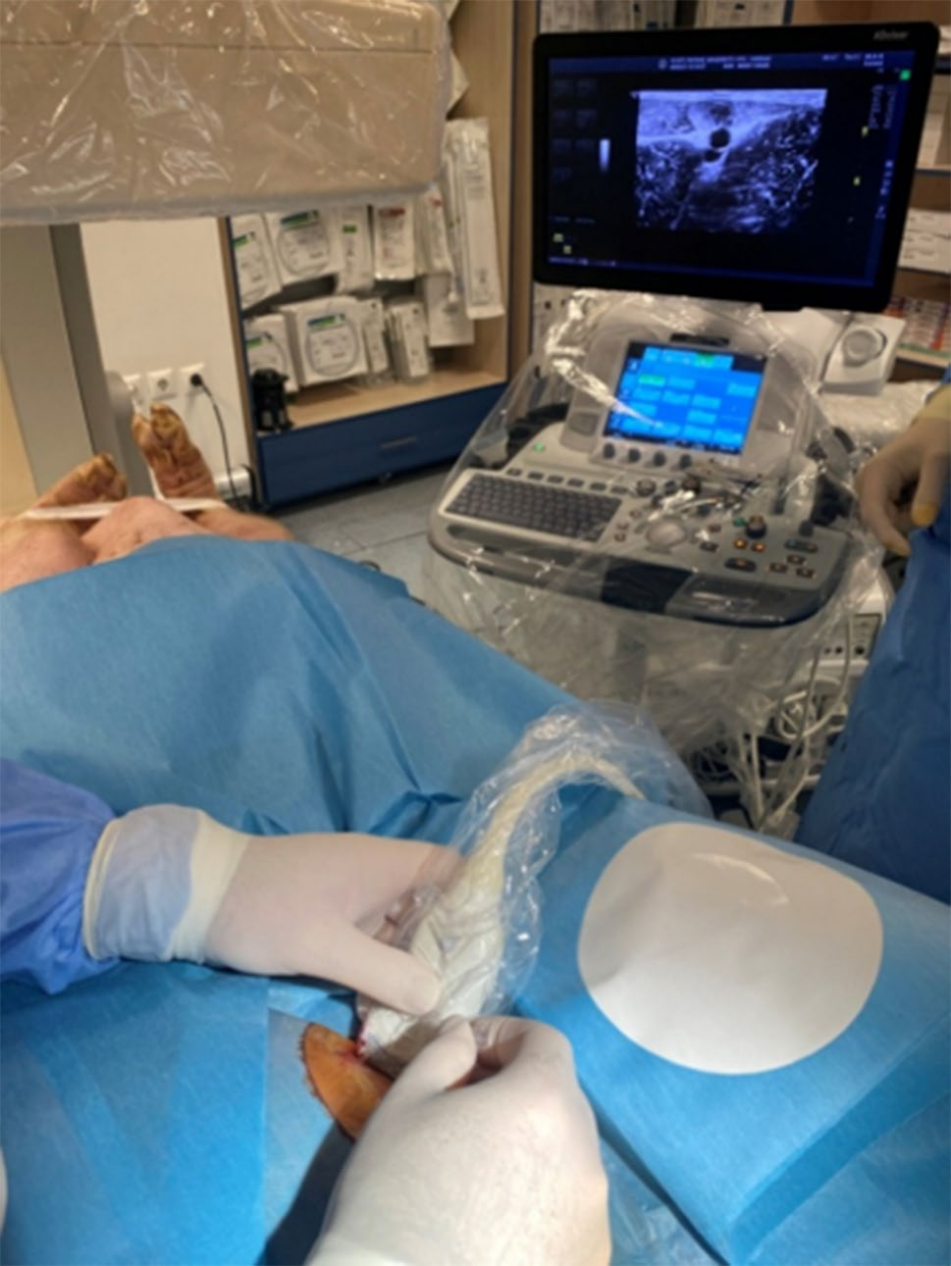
Ultrasonography-guided puncture of the right SFA.
